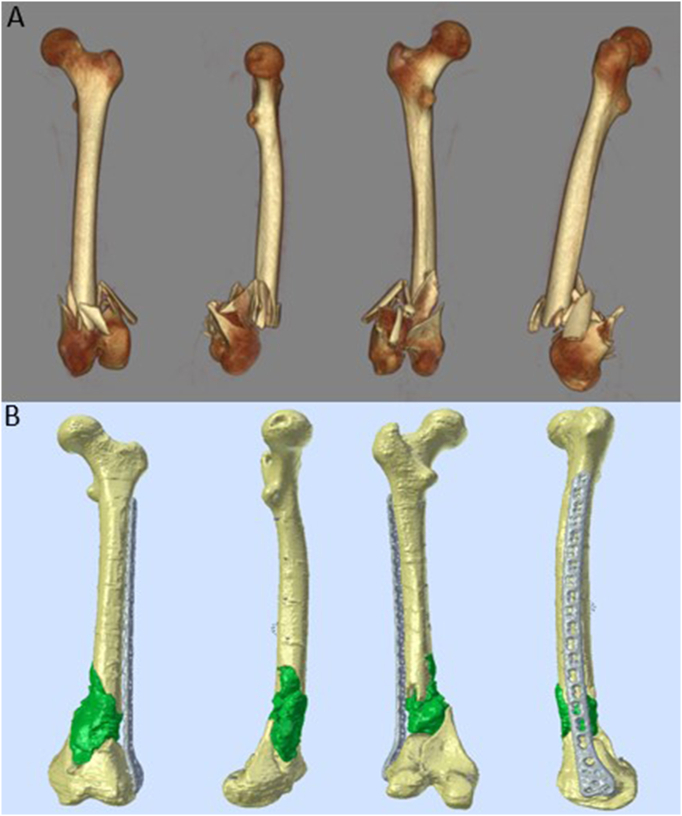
(A) The reconstruction was performed with the hospital's Picture Archiving and Communication System (PACS) after CT scan of the left femur. The distal dia-metaphyseal region has a severely comminuted and displaced fracture with articular extent (AO-classification 33C2.3). (B) 3D-reconstructed model of the femur from CT-data after initial fixation. A lateral condylar bridging plate is in place to stabilize the femur. The green surface indicates the circumferential defect that was filled with bone cement.
